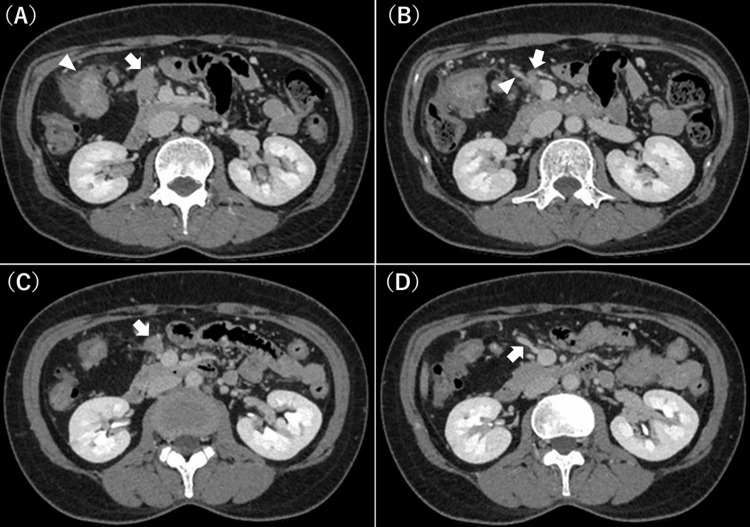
Computed tomography findings at the first visit (A, B) and after preoperative chemotherapy (C, D) (A) At the first visit, bulky regional lymph nodes (arrow) are in contact with the pancreatic head. The primary tumor is shown in the right transverse colon (arrowhead). As shown, the primary tumor is circumferential, and the colonoscope could not pass through the lesion. (B) Lymph nodes (arrow) are also in contact with the superior mesenteric vein (SMV) invading the root of the gastrocolic trunk (GCT) (arrowhead). (C) After chemotherapy, a thin fat layer appears between the shrunken lymph nodes (arrow) and the pancreatic head or SMV. (D) The root of the GCT (arrow), which should be sacrificed for en bloc lymph node dissection, is able to be encircled
